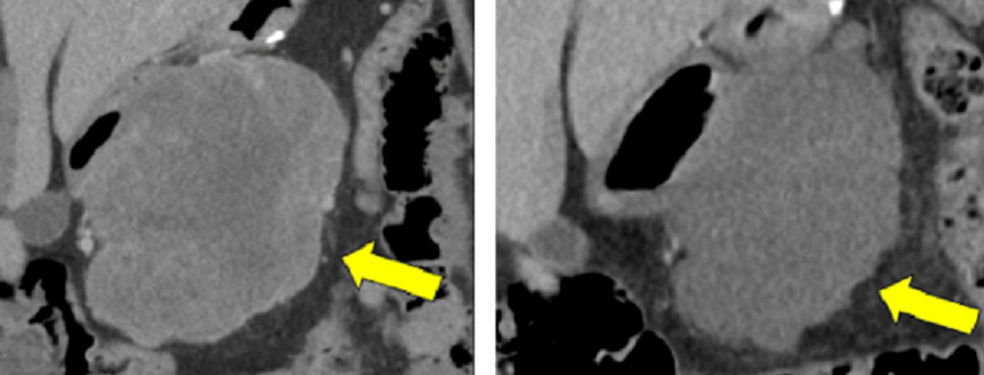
CT Abdomen of Recurrent Gastrointestinal Stromal Tumor (GIST) Before (Left) and After (Right) Second Course of Imatinib Therapy
